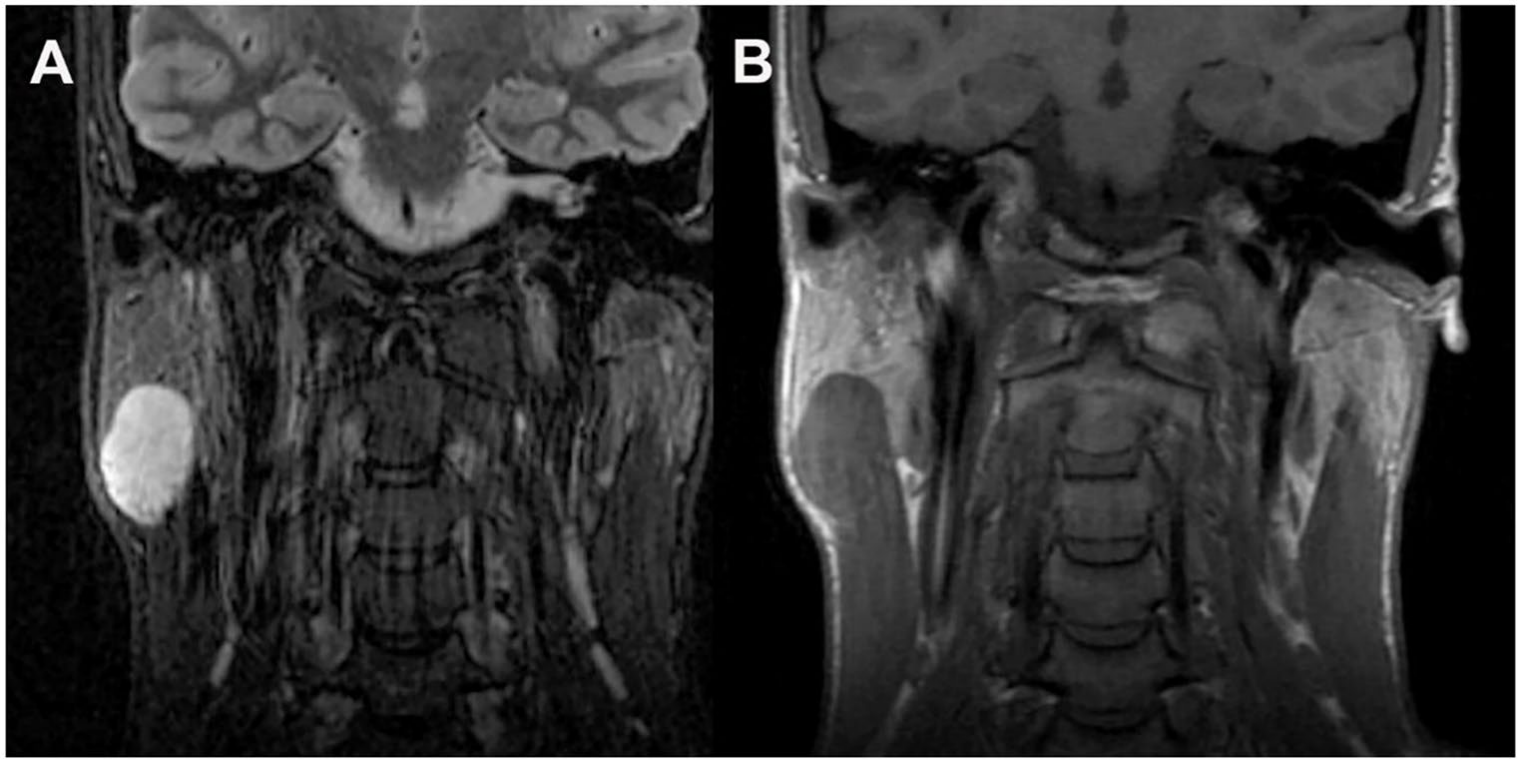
Coronal MR imaging of T2 hyperintense parotid tail mass. A) T2 (FSE STIR). B) T1
